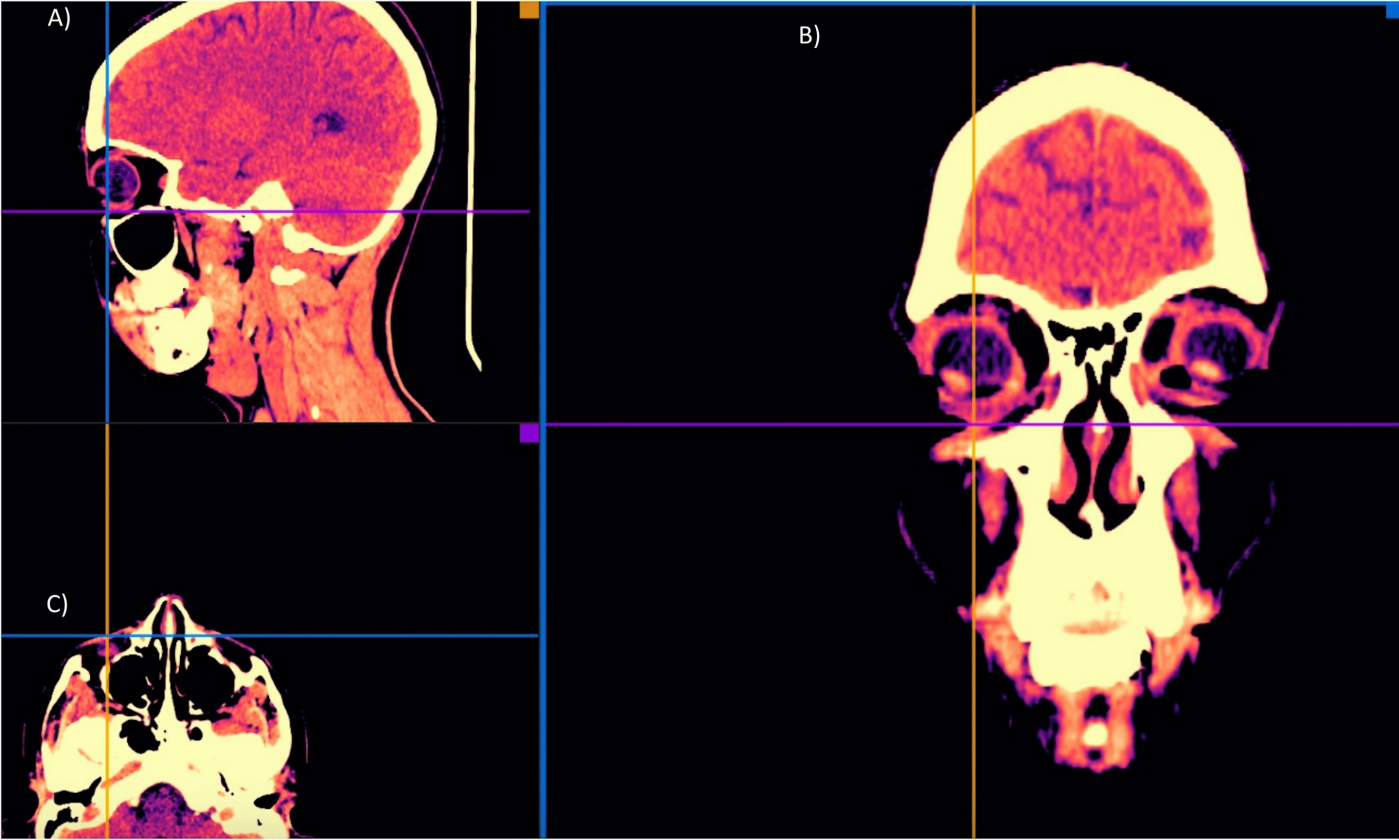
Facial CT multiplanar reconstruction (MPR) left lacrimal sulcus or multiplanar reconstruction. In these images it was possible to demonstrate in the three planes of space through a sagittal (A), coronal (B) and axial (C) view that Juvelook did not alter the tissue density or radiological CT pattern of the tissues after having been applied (two weeks post injection).
